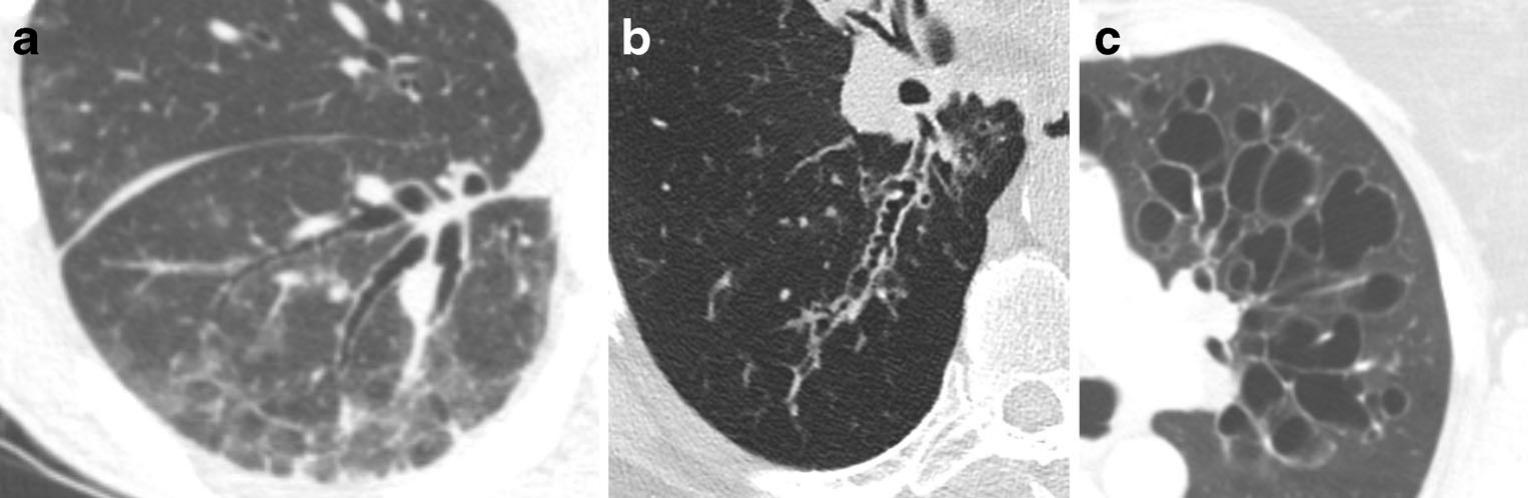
Types of bronchiectasis. Axial CT imaging of three different patients demonstrating the bronchiectasis types. a Cylindrical-type bronchiectasis is characterised by uniform nontapering or gradually tapering bronchial dilatation. b Varicose-type bronchiectasis is characterised by a beaded or “string-of-pearls” appearance with areas of alternating bronchial narrowing and dilatation. c Cystic-type bronchiectasis is characterised by markedly dilated cyst-like bronchi, often extending to the pleural surface with a “cluster-of-grapes” appearance
